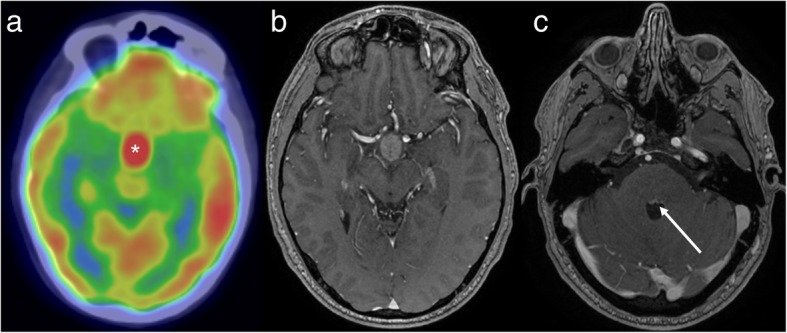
FDG-PET (a) in a patient with systemic lymphoma shows abnormal intracranial uptake (asterisk), consistent with secondary CNS involvement. This is localised to the hypothalamus on the post-contrast MRI (b). The post-contrast MRI (c) also demonstrates more extensive leptomeningeal disease than is appreciable on PET, including along the ependymal surface of the fourth ventricle (arrow)
